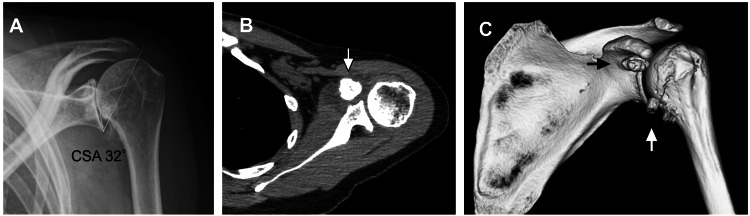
Initial radiograph and computed tomography scans of the patient's left shoulder (A) Radiograph shows the glenohumeral joint osteoarthritis and a critical shoulder angle of 32 degrees; (B) Computed tomography scans show bone fragments (arrow); (C) Three-dimensional computed tomography scans show bone fragments (arrow).
